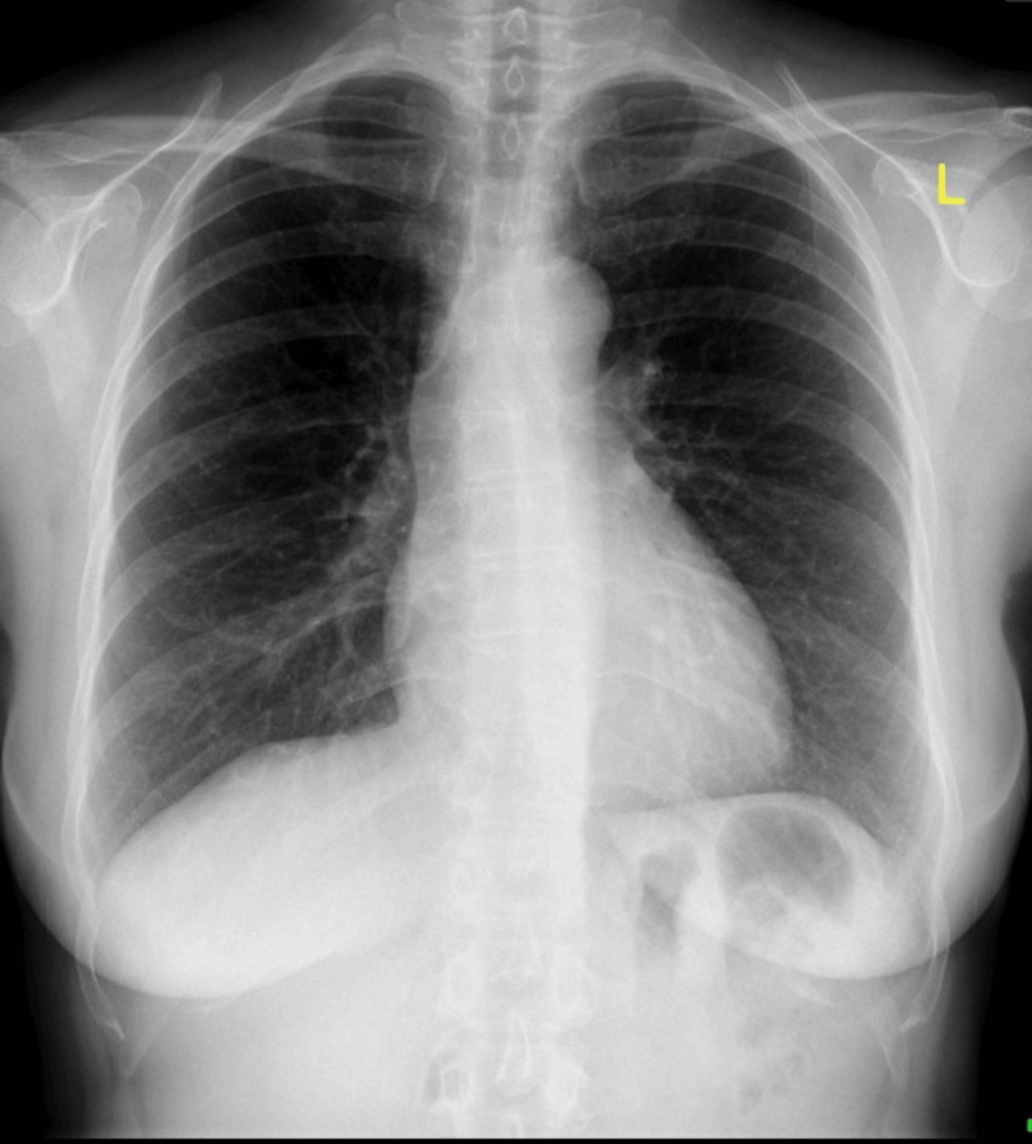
Chest X-ray with no marked findings
